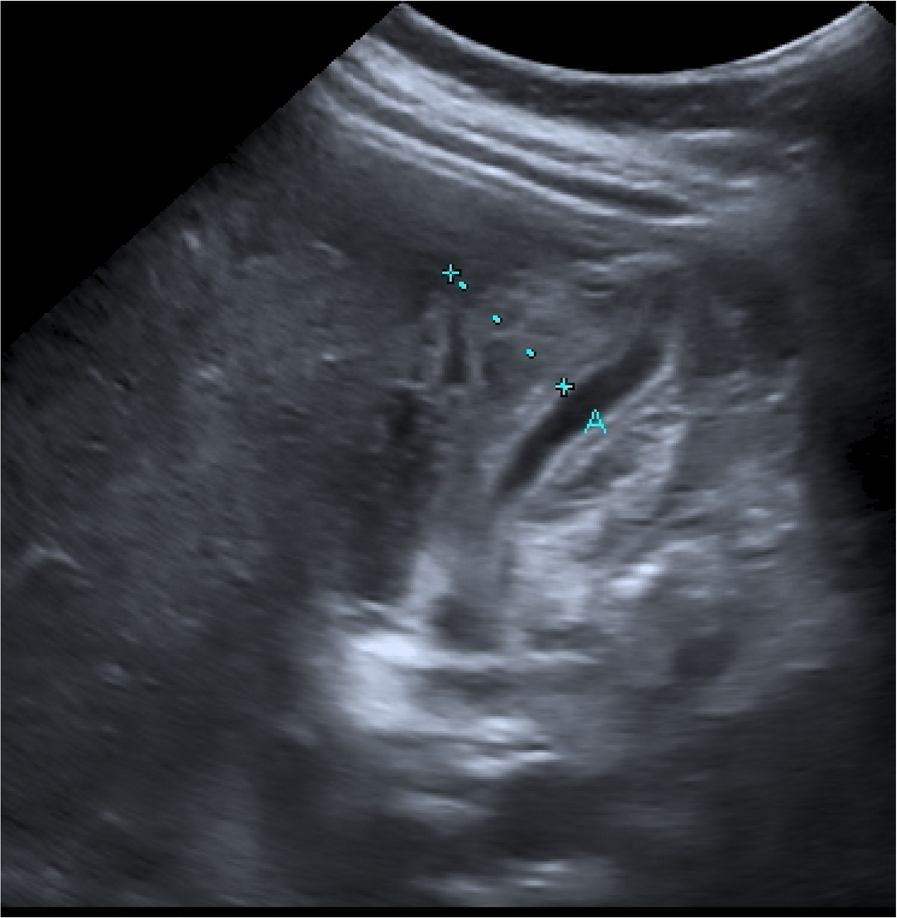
Transabdominal Ultrasound of the Gallbladder at the onset of abdominal pain. Distance A: 21 mm. Toshiba Aplio 500
